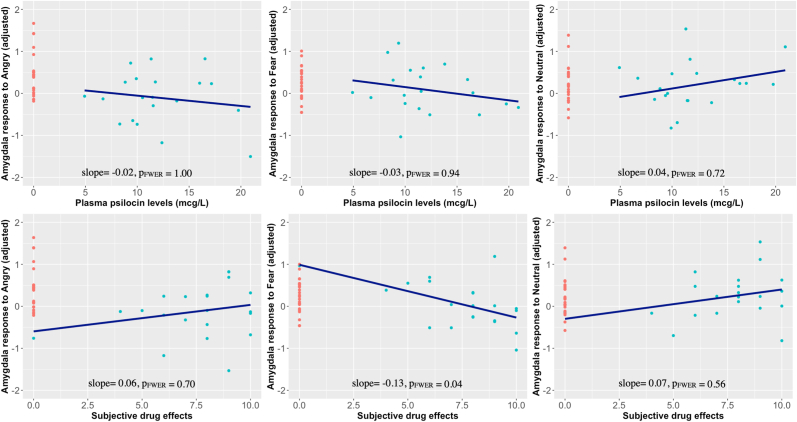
Plots of the amygdala response to angry, fearful and neutral faces (contrasted to geometric shapes) by plasma psilocin levels (PPL)/subjective drug intensity (SDI). Associations were estimated using linear mixed effects models (LMM) with MRI scanner as a covariate. In the LMMs, two additional mean parameters were introduced, encoding the association between (i) scan time, i.e., baseline vs psilocybin, and (ii) mean centred PPL/SDI with amygdala response (i.e., baseline PPL/SDI values were set to 0 and, while the mean PPL/SDI was subtracted from individual PPL/SDI values from the psilocybin MRI). These parameters can be interpreted as (i) the change in amygdala response for a person with mean PPL/SDI and (ii) how the amygdala response changes along with PPL/SDI. Red dots correspond to PPL/SDI at baseline, and blue dots correspond to PPL/SDI at psilocybin. The blues lines correspond to the estimated association between amygdala responses and PPL/SDI. P_FWER_ = corrected for family-wise error rate using the Bonferroni method with three tests.
